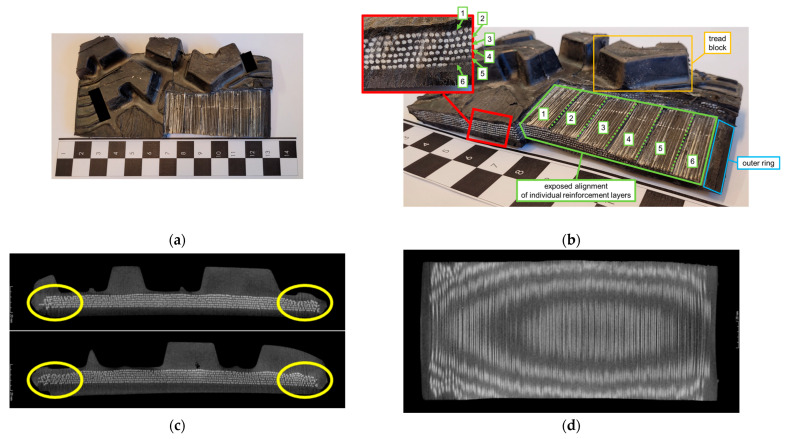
Portion of shear beam—NPT with 2D spoke: (a) view from the tread side with cut-outs, (b) identification: reinforcement layers; tread block; outer ring, (c) reinforcement layers (micro-computed tomography), (d) arrangement of the “threads” in the reinforcement layers (micro-computed tomography).
